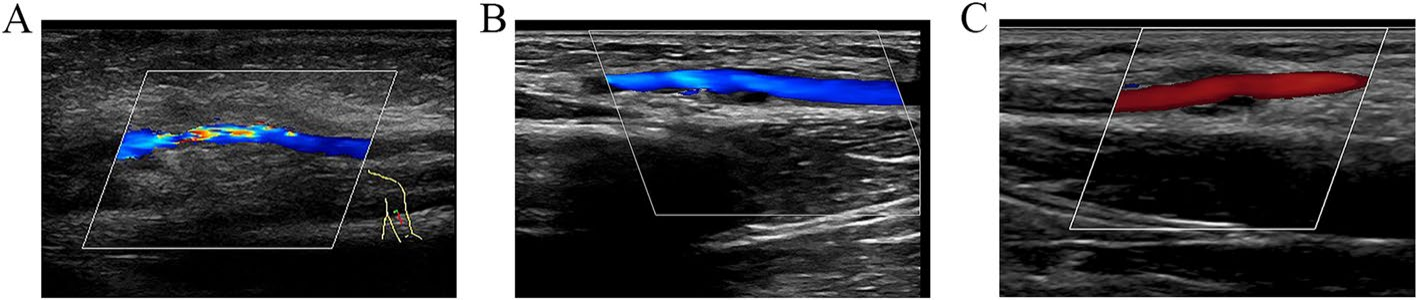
Postoperative Doppler ultrasonography results. A Partial stenosis and local inflammation around the reconstructed artery was seen at 1 week after surgery. B The artery stenosis and inflammation around the artery disappeared at 5 months. C Homogeneous and stable blood flow in the reconstructed brachial artery was seen without stenosis at 1 year
